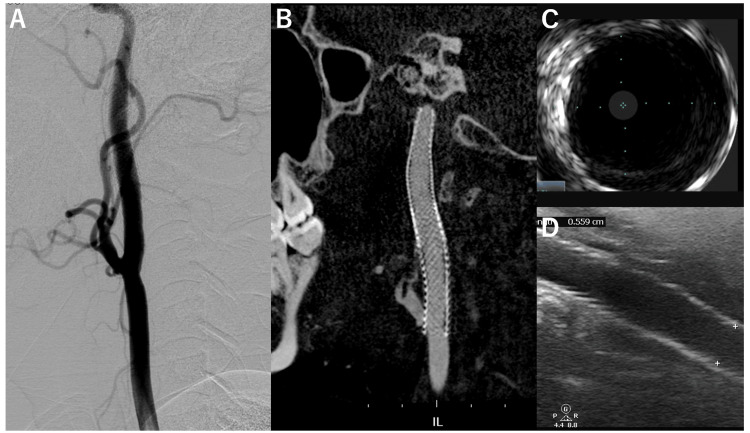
Imaging six months after the treatment. A: Lateral view of the left carotid artery. B: Lateral view in three-dimensional digital subtraction angiography of the left cervical carotid artery. C: Intravascular ultrasound of the left carotid artery. D: Trans-surface ultrasound image of the left carotid artery. No abnormalities were observed in these examinations.
